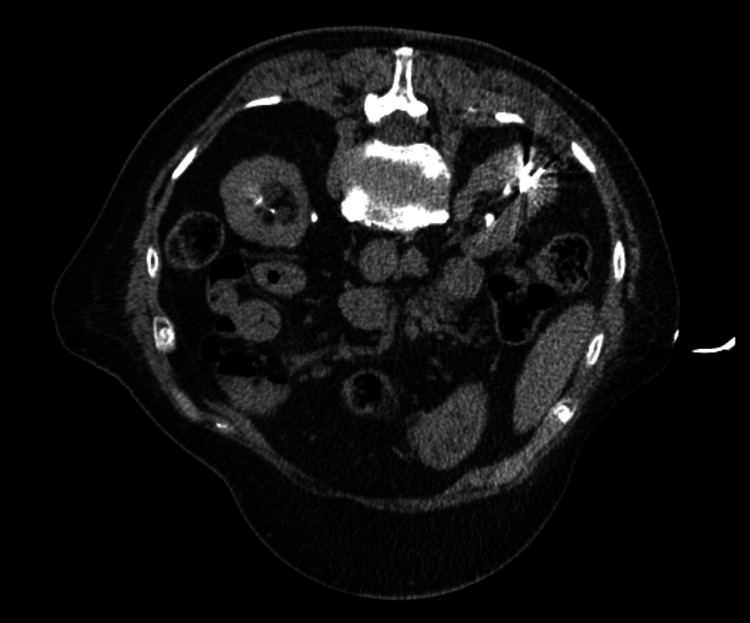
Axial CT demonstrating the ablation probes in the lesion during ablation
